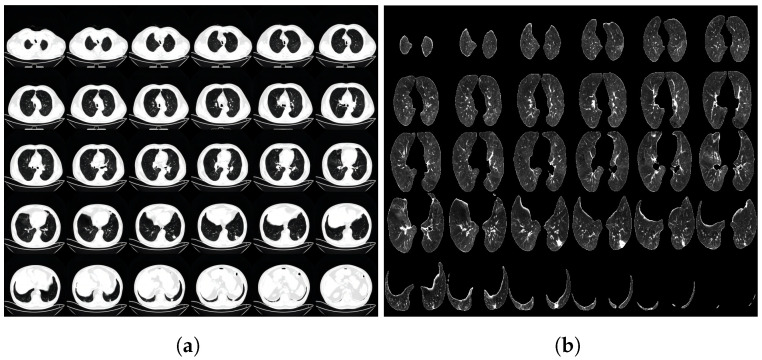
The CT image data before and after preprocessing, (a) is before preprocessing and (b) is after preprocessing.
